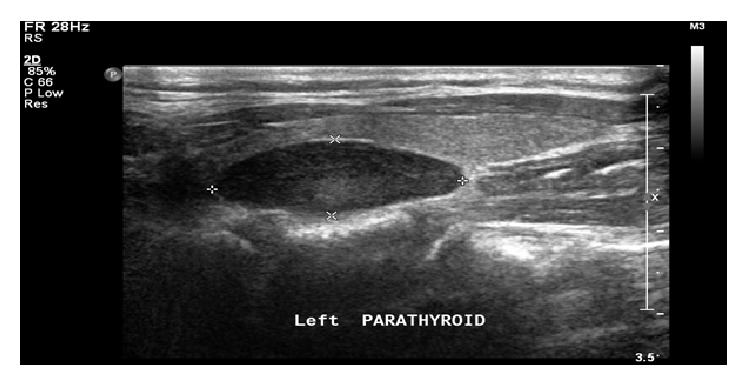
A well-defined, oval shaped, hyperechoic lesion identified in the inferior aspect of the left thyroid lobe exhibits mild-to-moderate internal vascularity on Doppler interrogation. It measures about 2.3 × 1 × 0.8 cm suggestive of parathyroid adenoma.
